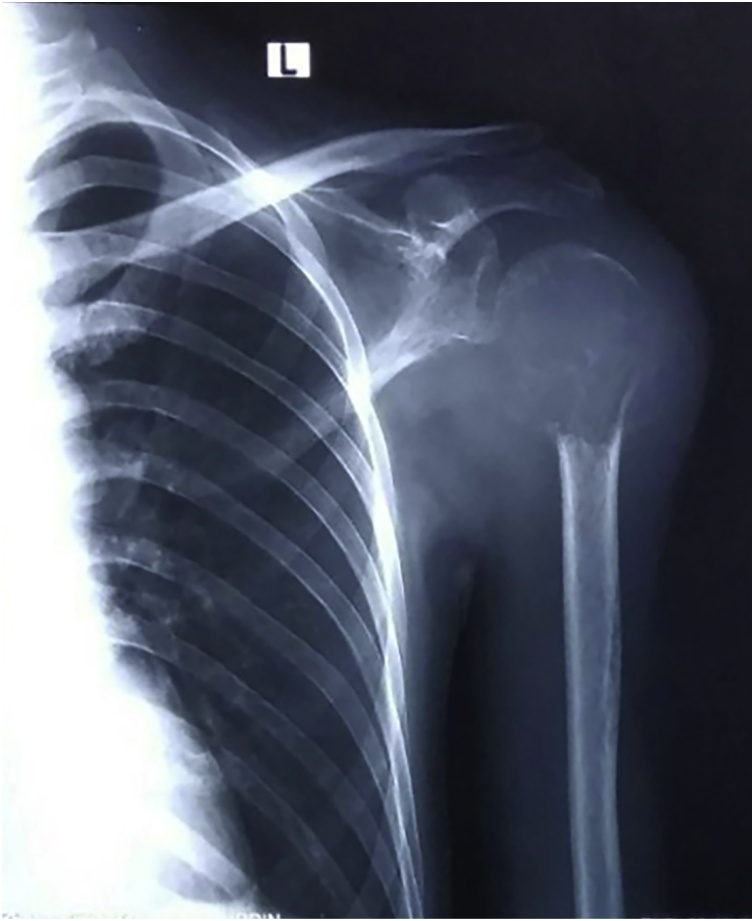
Radiograph of the left shoulder. An expansile and osteolytic lesion was visible with thinning of cortex in the proximal humerus.
